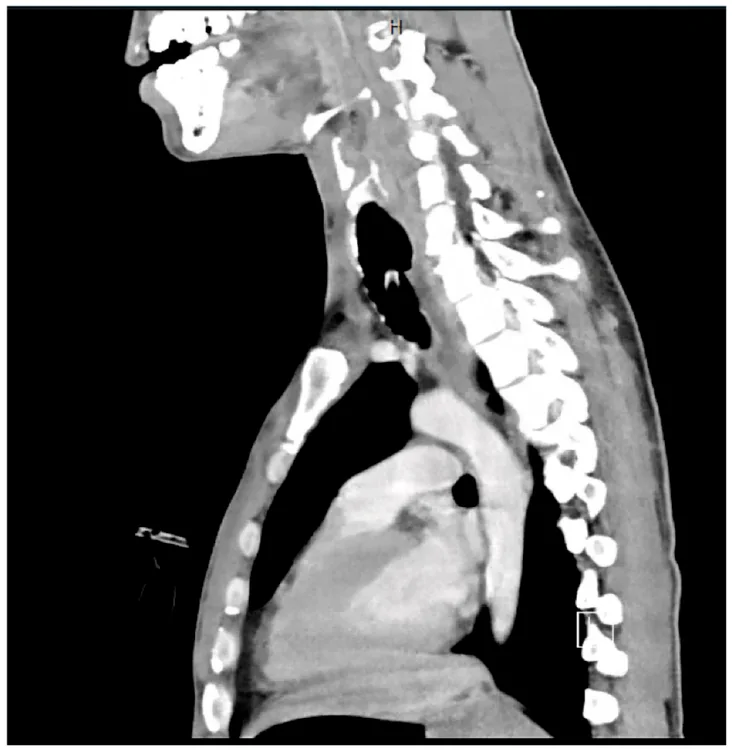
Contrast-enhanced sagittal CT image following the motor vehicle collision in Case 2, demonstrating extensive traumatic soft tissue injury involving the chest wall. The complete CT examination demonstrated a large right lateral and posterior chest wall hematoma extending into the right axillary region and toward the root of the neck.
